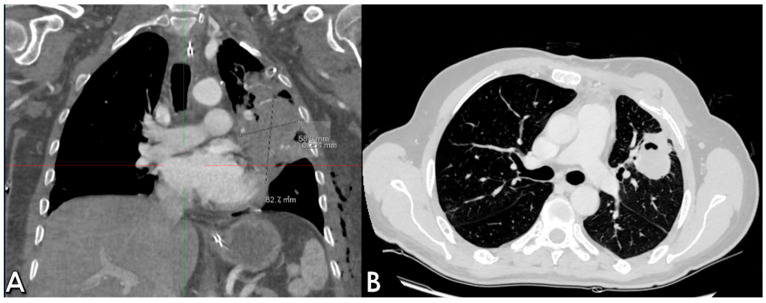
Postoperative sequential chest CT scans showing parenchymal LUL hematoma (A) and initial shrinking at CT follow-up (B).
